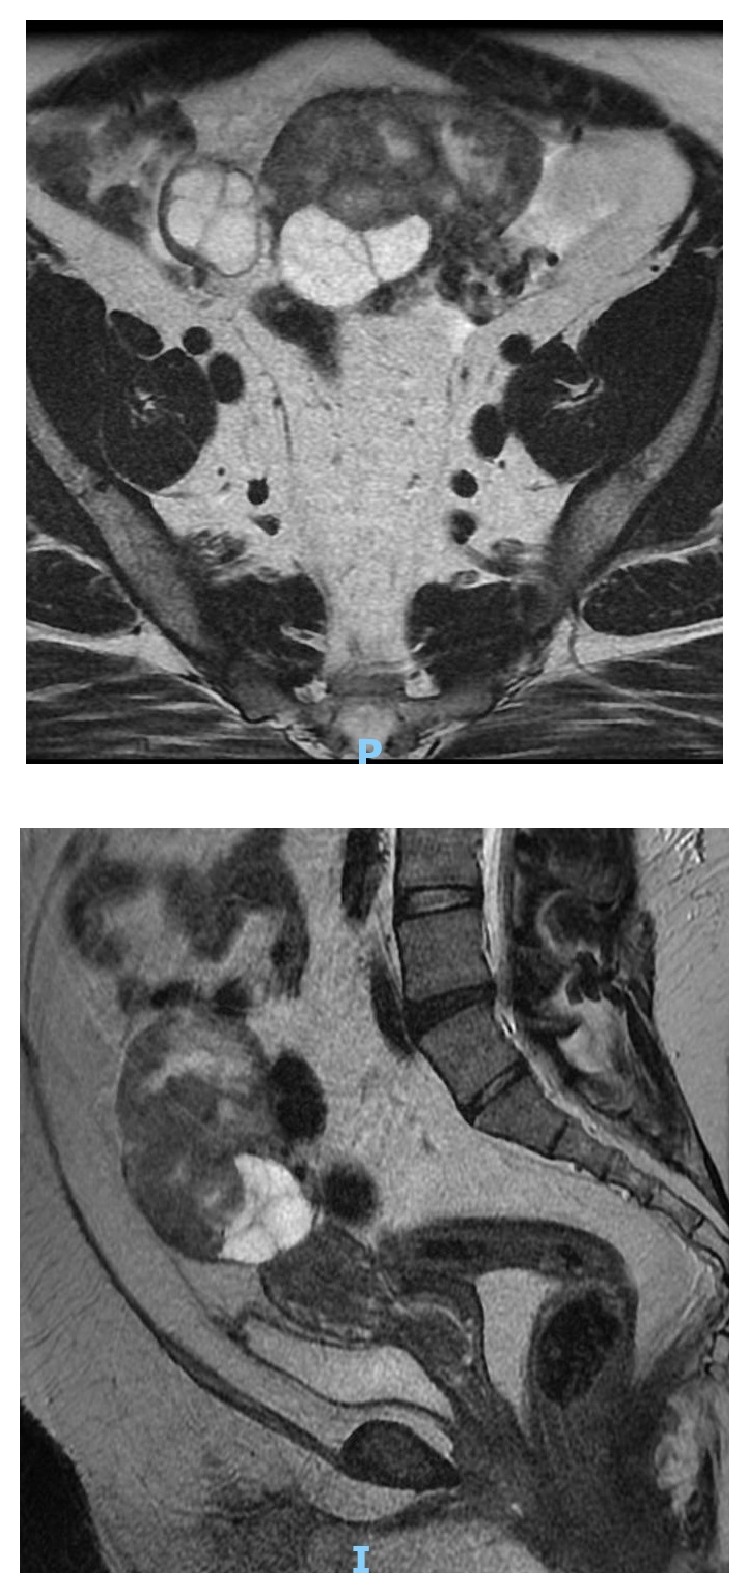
MRI abdomen and pelvis showed the presence of a large heterogeneous predominantly solid mass arising from the left ovary with cystic areas within. The solid components showed restricted diffusion and avid contrast enhancement. The mass was measured 8.4 x 6.1 x 8.9 cm. The solid components were hyperintense to muscle on T2w imaging and iso/hypointense on T1w imaging.
